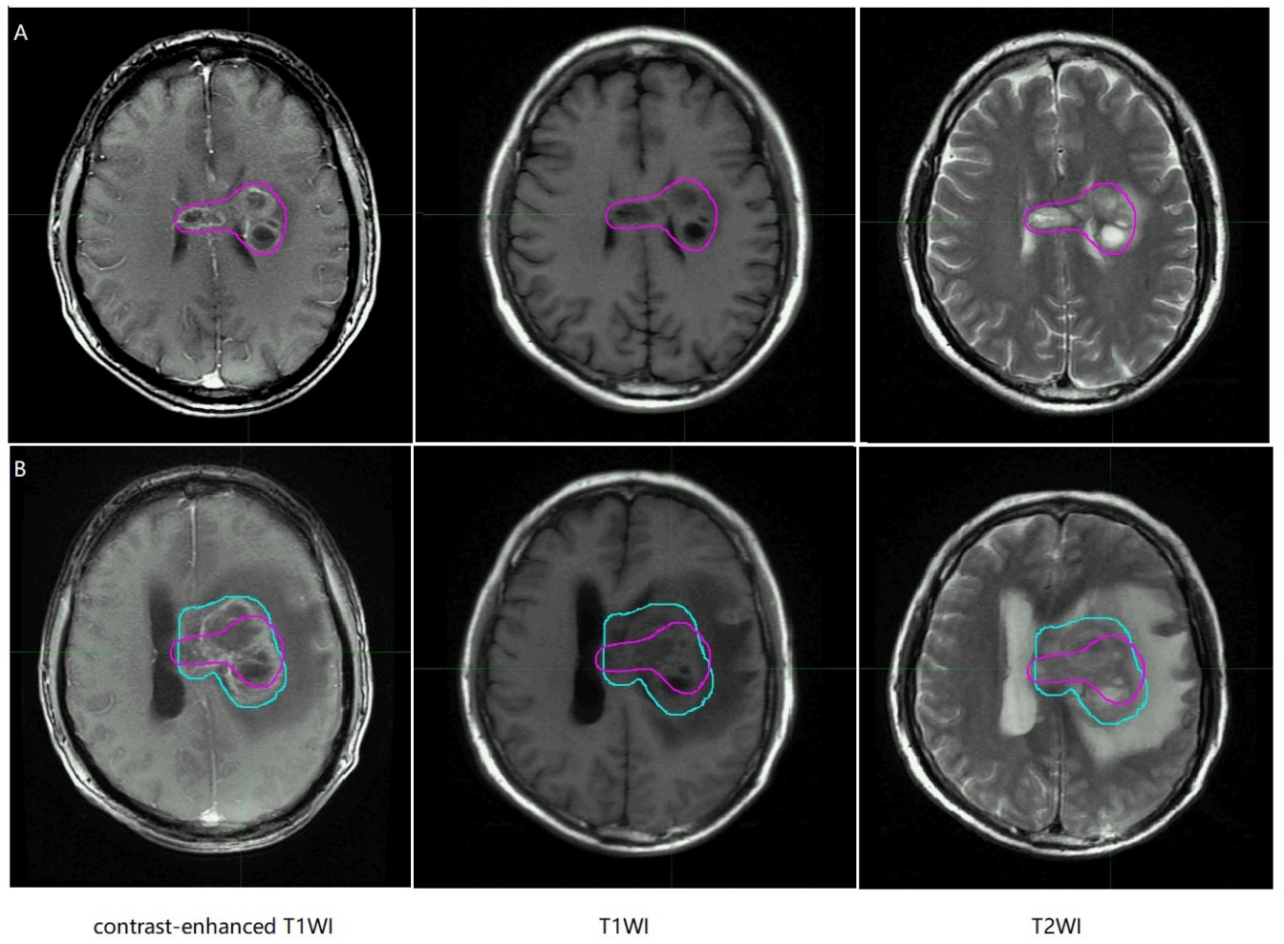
Representative recurrence patient's regular brain MRI images, the purple line and the blue line represent gross tumor volume of primary tumor and gross tumor volume of recurrent tumor. T1WI, T1 weighted image; T2WI, T2 weighted image.
